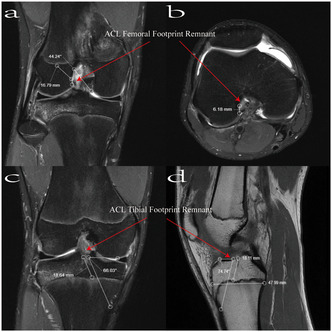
Using magnetic resonance imaging (MRI) of a right knee with an anterior cruciate ligament (ACL) rupture as an example: (a,b) are used to guide the creation of the femoral bone tunnel. (a) Measurement on coronal T1‐weighted MRI: The angle formed between the medial aspect of the lateral femoral condyle and a line parallel to the ACL fiber bundle (approximately 44.24°) is measured. This angle is used to guide the direction for creating both the minimally invasive tunnel and the graft tunnel on the femoral side. Additionally, the distance from the center of the femoral ACL footprint to the physeal line (16.79 mm) is measured. This distance determines the length of the graft tunnel on the femoral side, ensuring it stops before reaching the physis. (b) Measurement on axial T1‐weighted MRI: The distance from the center of the femoral ACL footprint to the posterior wall of the lateral femoral condyle (6.18 mm) is measured. This distance guides the selection of the appropriately sized femoral aimer. (c,d) are used to guide the creation of the tibial bone tunnel. (c) Measurement on coronal T1‐weighted MRI: The angle between the long axis of the tibia and a line parallel to the ACL fiber bundle (90°‐66.03° = 23.97°) is measured. This angle guides the placement angle of the tibial aimer in the sagittal plane. Furthermore, the distance from the center of the tibial ACL footprint to the physeal line (18.64 mm) is measured in the coronal plane. This distance dictates the length of the graft tunnel on the tibial side, ensuring it stops before reaching the physis. (d) Measurement on sagittal T2‐weighted MRI: The angle between the tibial plateau horizontal line and a line parallel to the ACL fiber bundle (74.74°) is measured. This angle guides the placement angle of the tibial aimer in the horizontal (axial) plane. Additionally, the distance from the center of the tibial ACL footprint to the physeal line (18.11 mm) is measured in the sagittal plane. This distance is used to define the length of the graft tunnel on the tibial side, ensuring it stops before reaching the physis.
